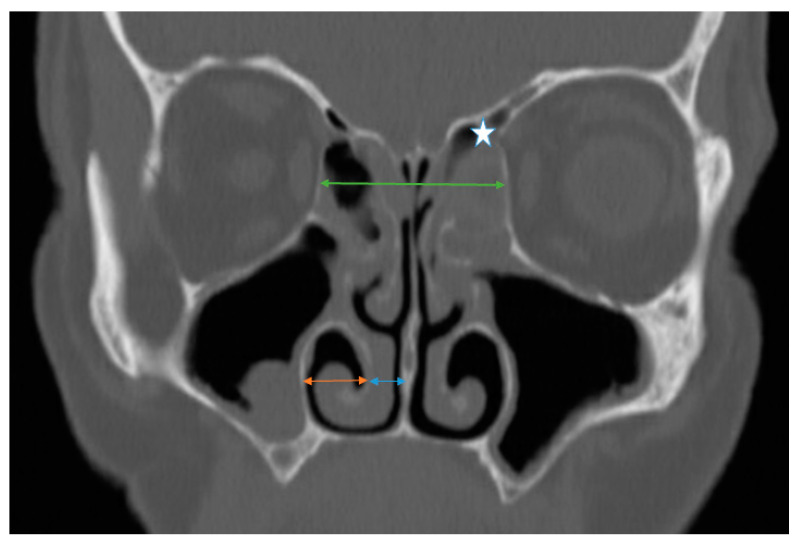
Sinus computed tomography scan, coronal plane. Star: the left anterior ethmoid artery. Green line: IOD in the coronal plane (IOD_Coronal_). Orange line: distance between the lateral border of the inferior turbinate to the lateral nasal wall (IT_L_). Blue line: distance between the medial border of the inferior turbinate and the septum.
